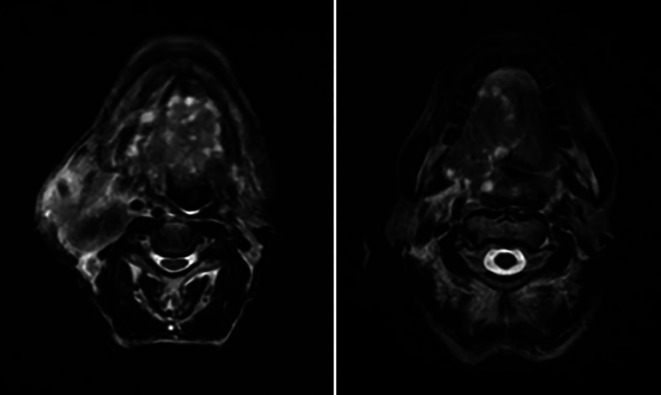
MRI (tongue) showing features mentioned above.
